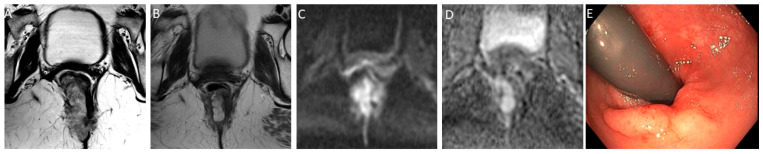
A 57-year-old female diagnosed with mucinous rectal carcinoma. Initial T2-weighted oblique axial image reveals a large low mucinous rectal carcinoma invading the external sphincter (A). Following total neoadjuvant therapy, a considerable decrease in tumor size was observed about four months later, with some mucin deposits persisting (B). While the intermediate T2 signal has diminished, indicative of a response to treatment, slender strands of fibrosis remain. b-800 diffusion-weighted imaging (C) and apparent diffusion coefficient (ADC) mapping (D) are somewhat compromised in their diagnostic utility by the ‘T2 shine-through” effect associated with mucin. Furthermore, endoscopic examination identified a healed ulcer with overlying irregular mucosa in the posterior anal canal (E).
